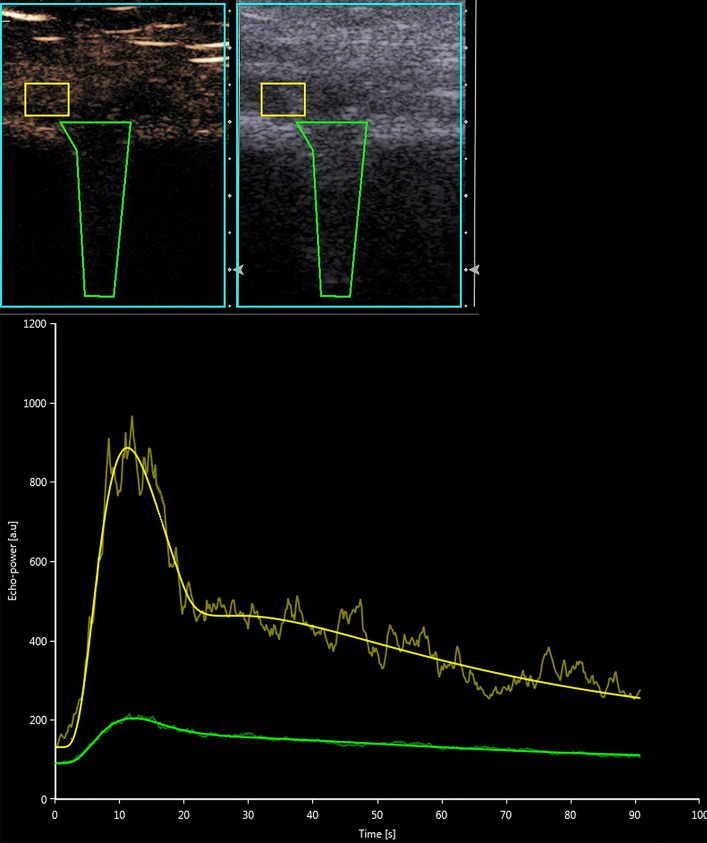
Contrast-enhanced ultrasound (CEUS) examination and evaluation of an osteotomy gap (patient without ABG). Upper part: sonography of the osteotomy gap with the specific contrast-enhanced cadence mode (left) and the standard b-mode (right). Lower part: evaluation over time of both regions of interests (ROI) with the dedicated quantification software VueBox (Bracco Imaging). Green: ROI 1 in the osteotomy gap. Yellow: ROI 2 in adjacent tissue without fascia or vessels (colour figure online)
